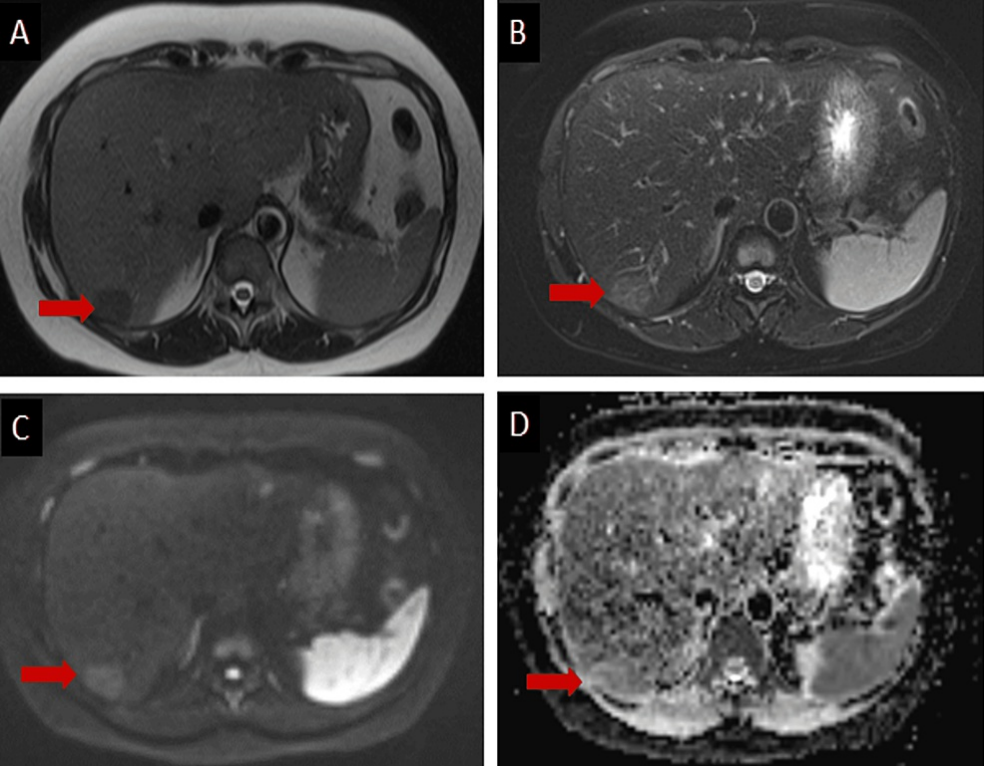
Multiple MRI sequences demonstrate the lesion in segment 6 (red arrows), which appears hypointense on T2-weighted image (A) and slightly hyperintense on T2 - weighted image fat saturation (B) with no diffusion restriction (C, diffusion-weighted images & D, ADC map).
